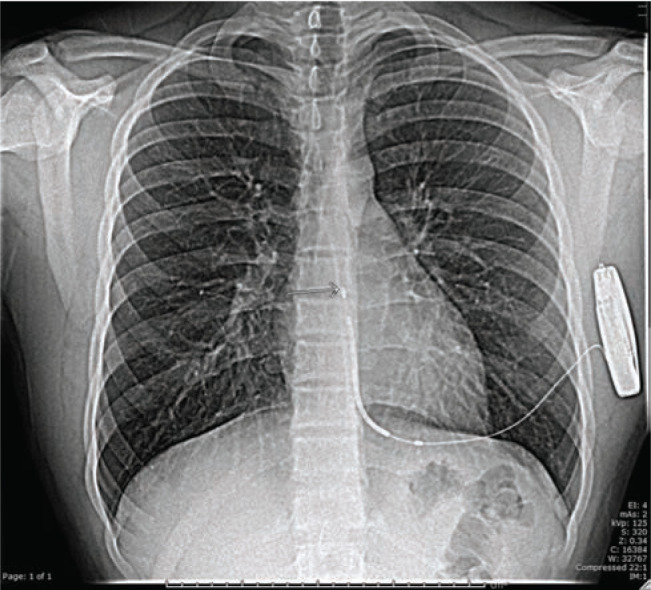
Chest X-ray showing lead migration with displacement of approximately 4.5 cm.
